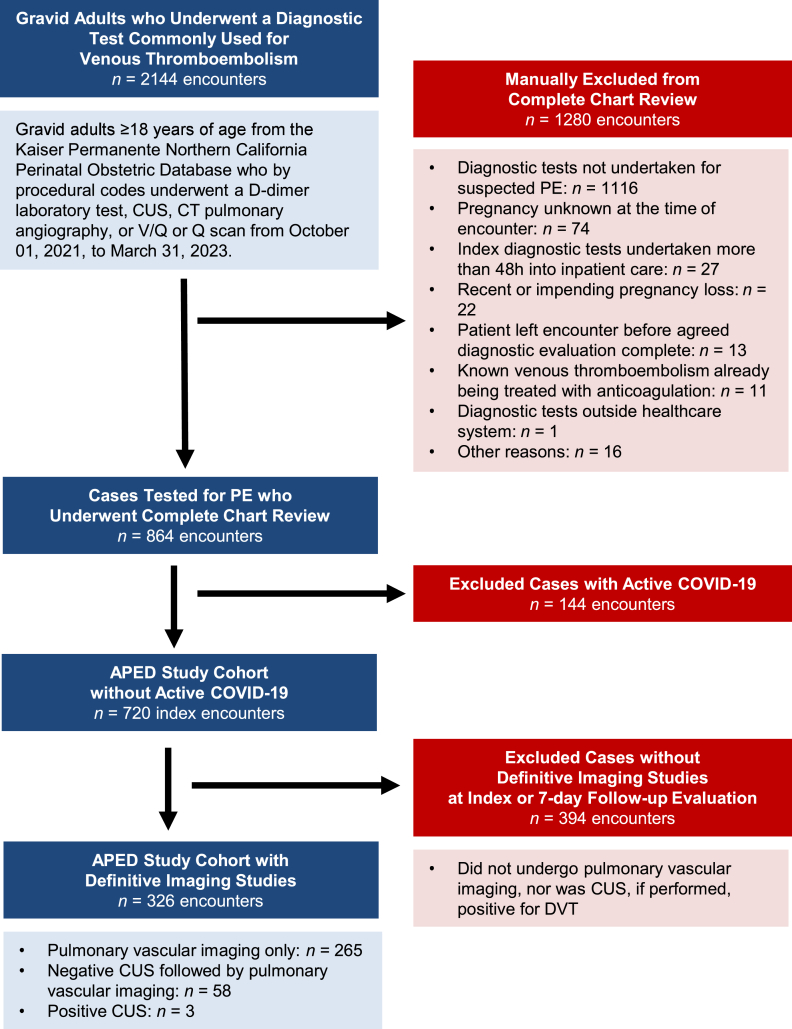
Cohort assembly. APED, Antenatal Pulmonary Embolism Diagnostics; CT, computed tomography; CUS, compression ultrasonography; DVT, deep vein thrombosis; PE, pulmonary embolism; V/Q, ventilation/perfusion.
